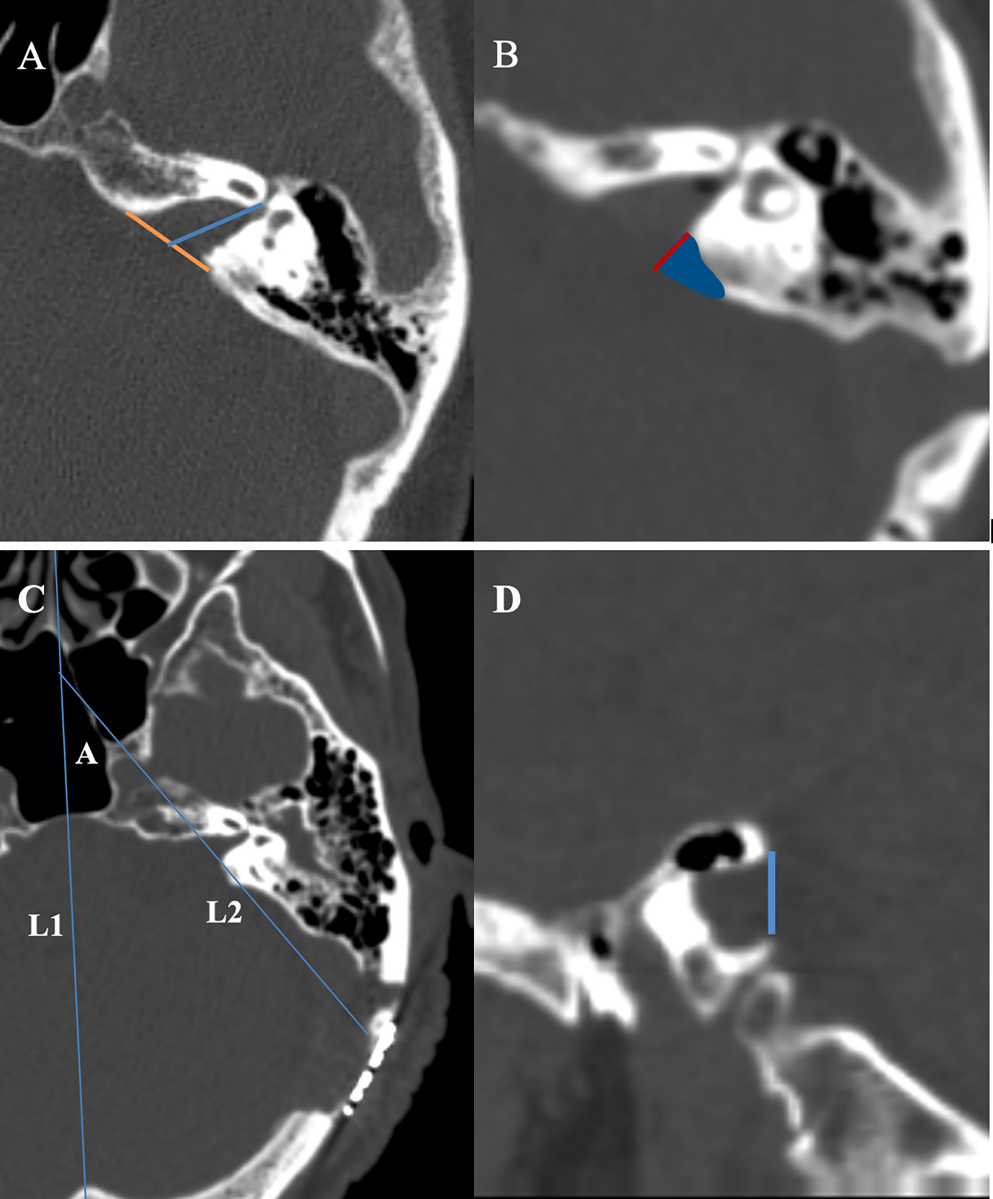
(A) Measurement of the absolute tumor extension into the internal auditory canal. On a thin slice CT scan, the slice with the most lateral extension of the tumor is selected for measurement. An auxiliary line (yellow) is drawn between the anterior and posterior lip of the porus acoustics. The length of absolute tumor extension is defined as the distance between the midpoint of the yellow line and the most lateral point of tumor extension. (B) We use Brainlab software to fuse the thin-slice CT scan images before and after surgery. The blue area shows the bone removal part of the internal auditory canal, and the length of the red line represents the exposure length of the internal auditory canal. (C) L1: the middle sagittal line at the axial plane; L2:the line of the bone removal plane of the posterior and lateral wall of the internal auditory canal at the axial plane; angle A the angle between L1 and L2 stands for the drilling angle. (D) The width of internal auditory canal exposure is defined as the distance as a blue line at the sagittal plane.
